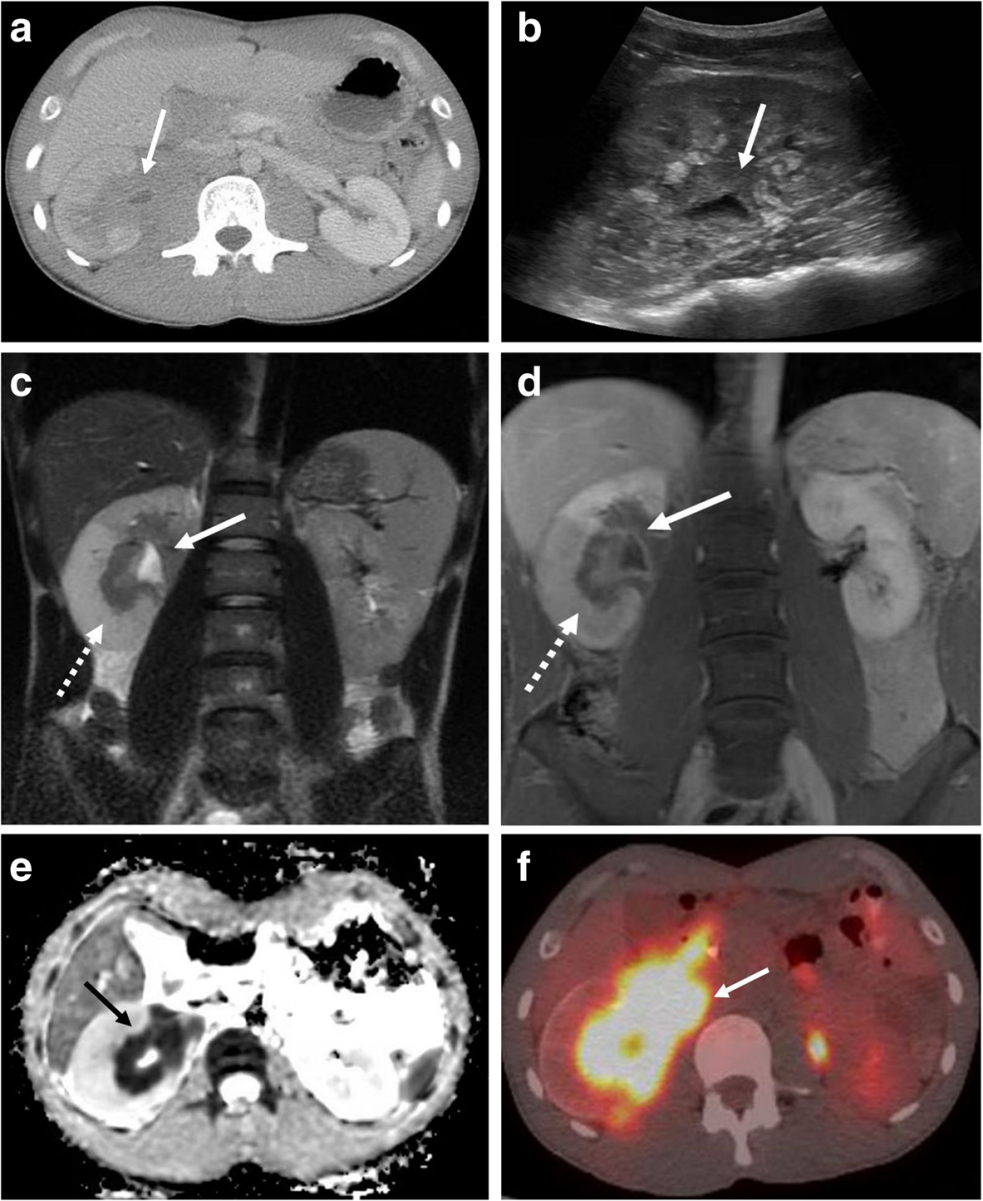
Renal collecting system involvement. A 24-year-old male presenting with abdominal and lower back pain. a Contrast-enhanced axial CT image demonstrates an infiltrative soft tissue mass (arrow) encasing the right renal collecting system with resultant dilated renal pelvis and urothelial thickening. b Corresponding sagittal grayscale ultrasound image (b) demonstrates obliteration of the normal renal sinus fat by hypoechoic tissue encasing the renal pelvis (arrow). Follow-up MRI with (c) coronal T2-weighted, (d) coronal T1-weighted post contrast, and (e) axial apparent diffusion coefficient images shows a right renal pelvis mass with associated diffusion restriction (solid arrows) with edema and delayed enhancement of portions of the right renal parenchyma (dashed arrows). f Follow-up fused axial PET-CT demonstrates associated hypermetabolic activity (arrow)
